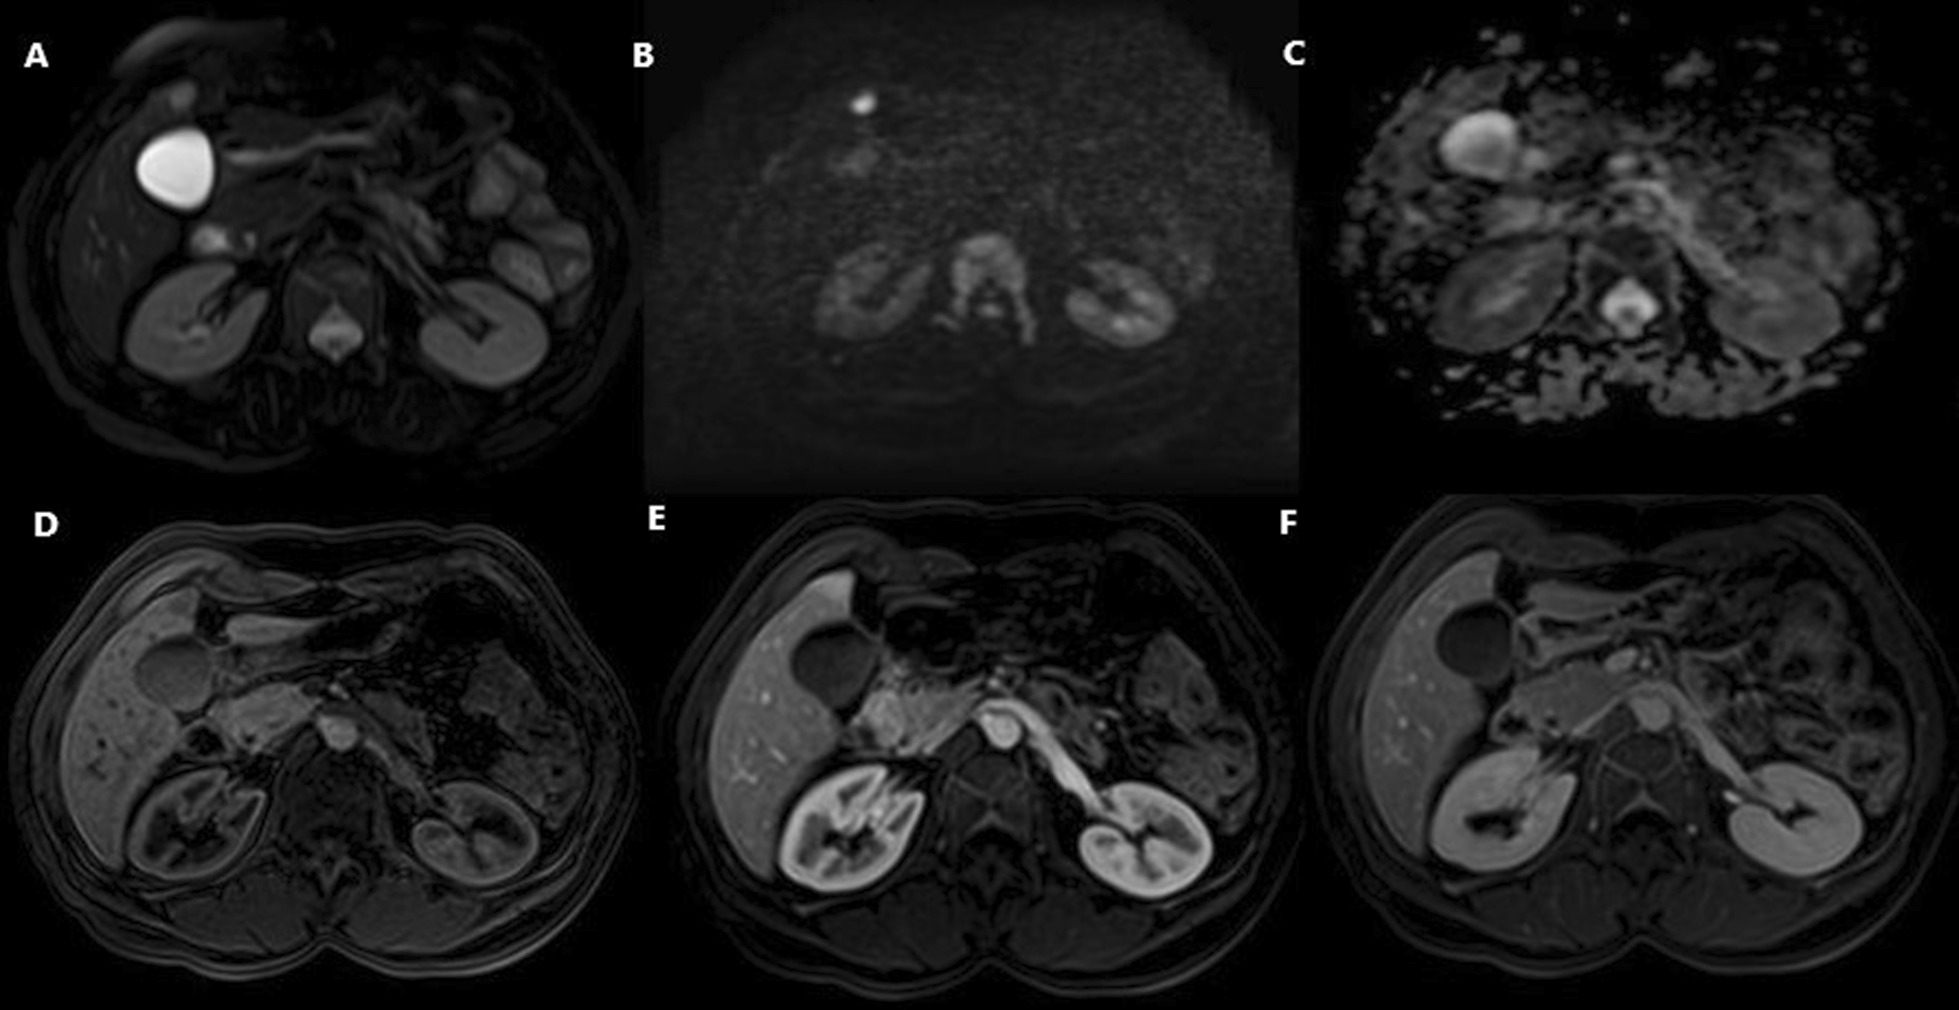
A–C MRI showing a well-defined lesion in IVB segment hyperintense T2 fat suppressed (A) demonstrating restriction to diffusion (B, C) with delayed enhancement on contrast-enhanced images (D–F).
